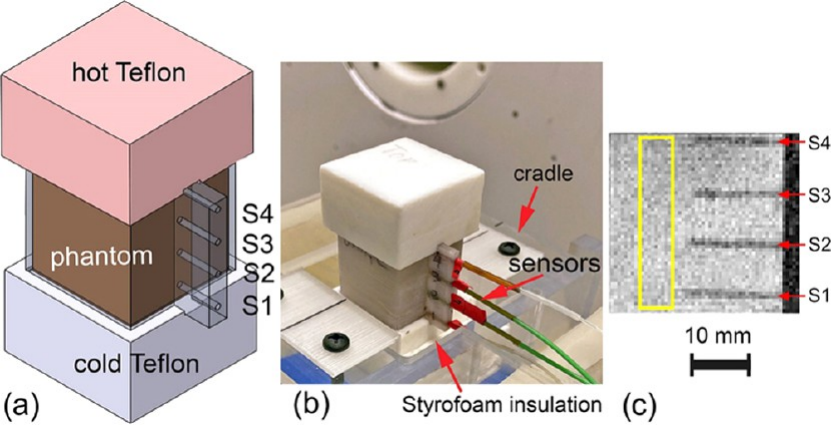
MR imaging in the presence
